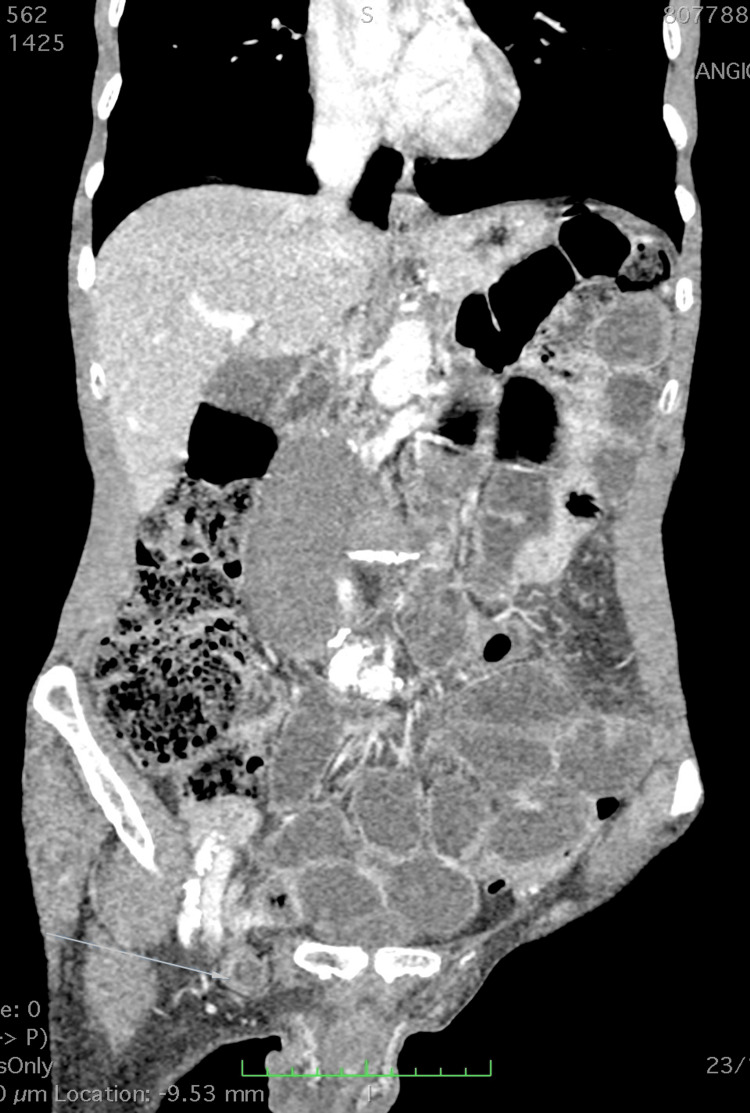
CT scan showing dilated bowel loops and an incarcerated femoral hernia
